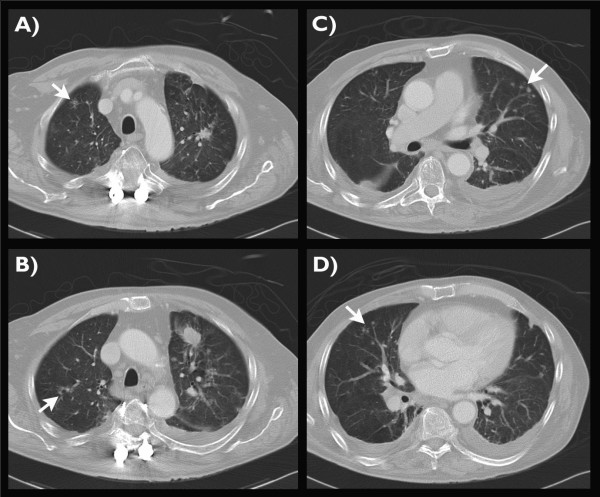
Follow-up contrast enhanced CT scan of the chest. The axial CT reconstructions in craniocaudal sequence (A-D) show exudative pulmonary tuberculosis with multiple novel opacities and tree-in-bud sign (arrows). Secondary findings: post-specific scarring (A), tuberculoma (B) of the left upper lobe, and small bilateral pleural effusions (B-D).
